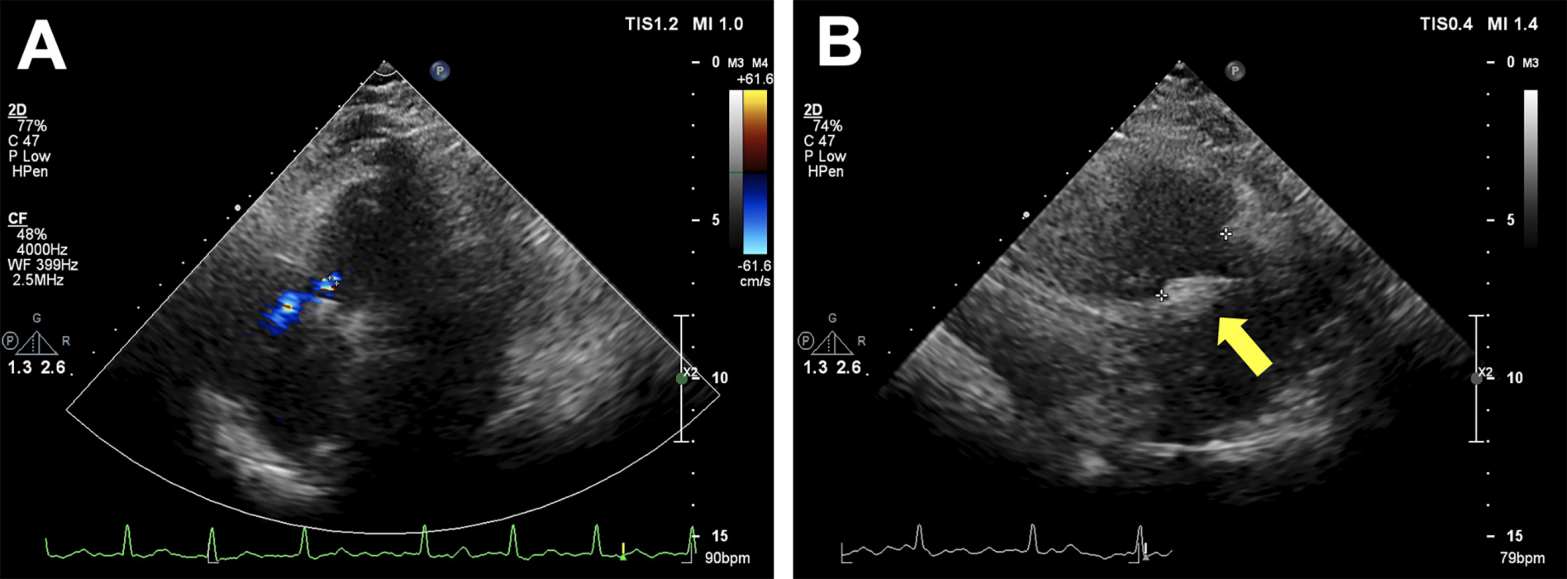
Transthoracic echocardiography after 3 months of follow-up. (A) Showing mild residual tricuspid regurgitation. (B) Showing the secure position of the device (yellow arrow).
